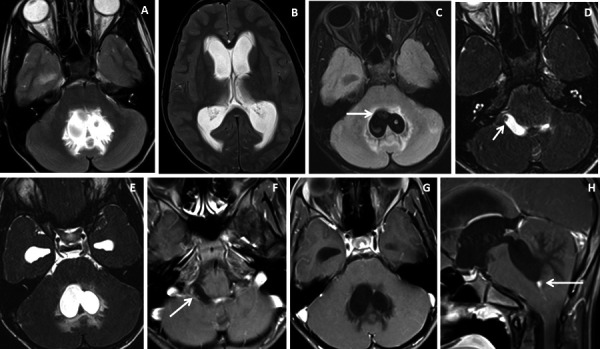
Brain magnetic resonance imaging of 13 years male with severe headache, vomiting and acute neurological deterioration. (A-B) Axial T2WI images show marked distension and dilatation of the fourth ventricle with marked periventricular CSF seepage and supratentorial hydrocephalus. (C) Axial FLAIR image shows cystic lesions within the fourth ventricle (arrow). (D-E) Axial heavily T2W images show thin-walled hyperintense cystic lesions within the 4^th^ ventricle with an oval to elongated appearing cystic lesion with scolex in the right foramen of Luschka (arrow). (F-H) Axial and sagittal T1W post-contrast image shows a thin peripheral enhancing cystic lesion with an irregular mural nodule in the right foramen of Luschka (arrow) and thin smooth enhancement of the intraventricular cysts. Nodular enhancement was seen in midline inferior margin of 4^th^ ventricle near to foramen of Magendie (arrow).
